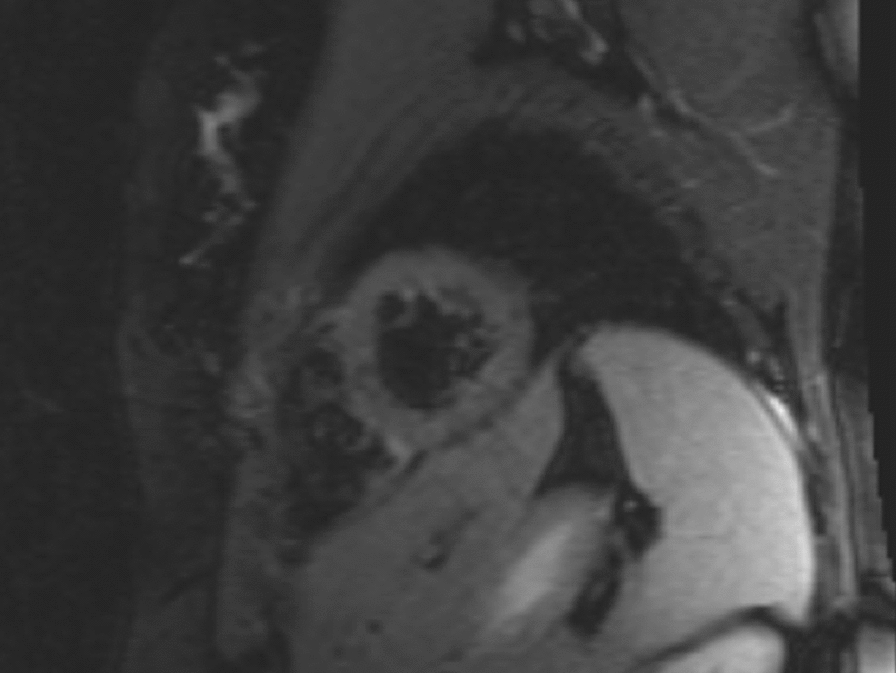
Resolution of prior oedema seen on cardiac MRI post vaccination with BNT162b2. Sagittal T2 STIR image of cardiac MRI of Case 2 patient prior to vaccination with NVX-coV2373, demonstrating complete resolution of previously observed oedema
